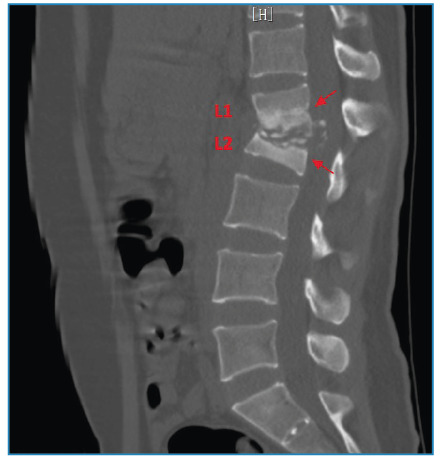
Computed tomography scan (sagittal section) showing apparent destruction of the L1 and L2 vertebrae, severe collapse of the L1 vertebra, and obvious kyphosis (type B) with involvement of the L1 and L2 vertebrae (red arrows).
